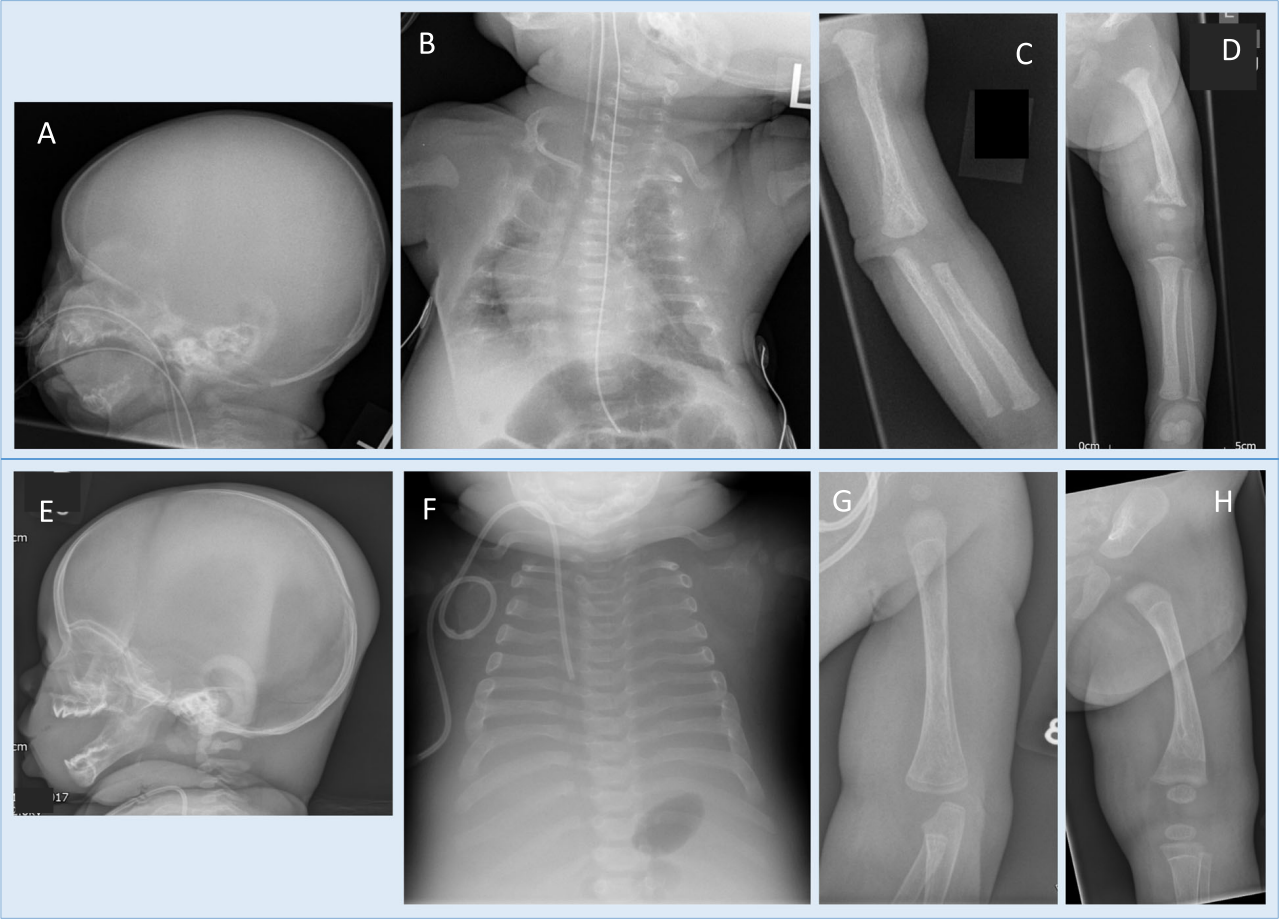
Radiological findings. Upper panel (a-d) shows early postnatal plain radiographs (Day 12) and lower panel (e-h) shows corresponding post-mortem plain radiographs. Bone density is considerably less in each upper panel image compared with lower images (skull a and e, chest b and f, upper limb c and g, lower limb d and h). Chest X-rays (b and f): thoracic size is extremely reduced (b), to a lesser degree post-mortem (f), which also shows broad and more calcified ribs, although with irregularities posteriorly and laterally consistent with healed fractures. Upper limb: early postnatal imaging (c) shows ill-defined metaphyses; then (g) more sharply defined metaphyses, prominent growth restart lines in diaphyses and bone-in-bone appearance. Lower limb: d shows initial poorly defined metaphyses, reduced bone density and metaphyseal fractures; then H shows healed fractures, better metaphyseal delineation, prominent growth restart lines in diaphyses and bone-in-bone appearance
